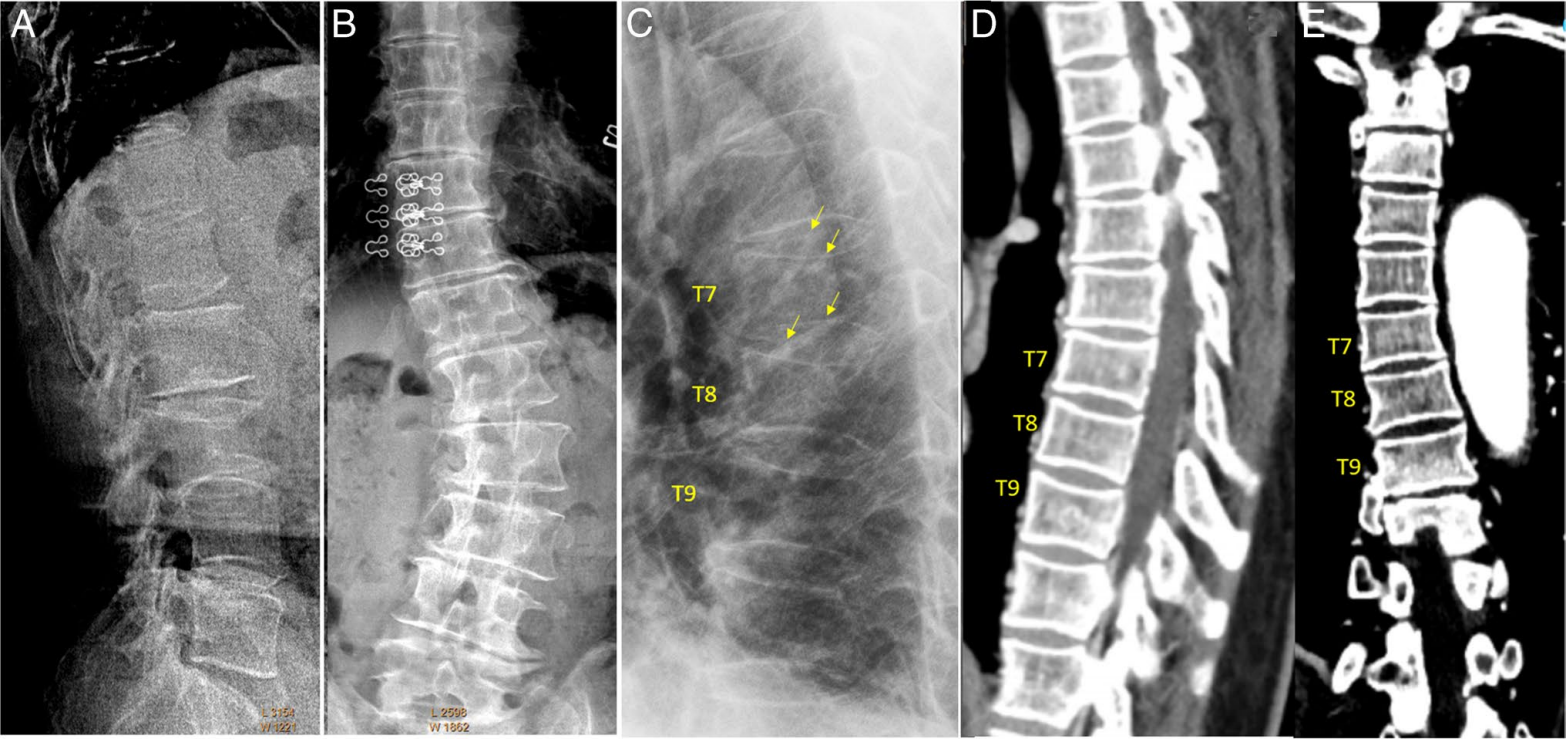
Scoliosis induced artifacts on spine lateral radiographs. These artifacts commonly affect a few adjacent vertebrae. A and B A case of lumbar spine scoliosis in an elderly woman. Lateral radiograph shows a mimicker of OLVF at L3 with “bean-can” appearances of the endplates. Frontal radiograph demonstrates lumbar spine scoliosis, however lumbar vertebrae appear to be of normal shape. C, D, and (E) Chest imaging of an elderly woman with thoracic spine scoliosis. Lateral radiograph (C) shows superior and inferior endplate “depressions” of T7, T8, and T9. Careful observation shows the endplate rings are projected as apparent ovals (“bean-can” appearance) suggesting “rotation” of the vertebrae relative to the expected position (or relative to X-ray beam). Reconstructed sagittal CT image (D) and coronal CT image (E) demonstrate slight dextroconvex thoracic scoliosis, involving the T6 to T9 vertebrae. There is no vertebral deformity demonstrated for the T7 to T9 vertebrae on images (D) and (E). Note the anterior vertebral heights of T7 to T9 appear maintained on (C). OLVF, osteoporotic-like vertebral fracture. A and B Reproduced with permission from Wáng. Quant Imaging Med Surg. 2022;12:3495–3514. C, D, and E Reproduced with permission from Du and Wáng. Osteoporos Int. 2022;33:1569–1577
